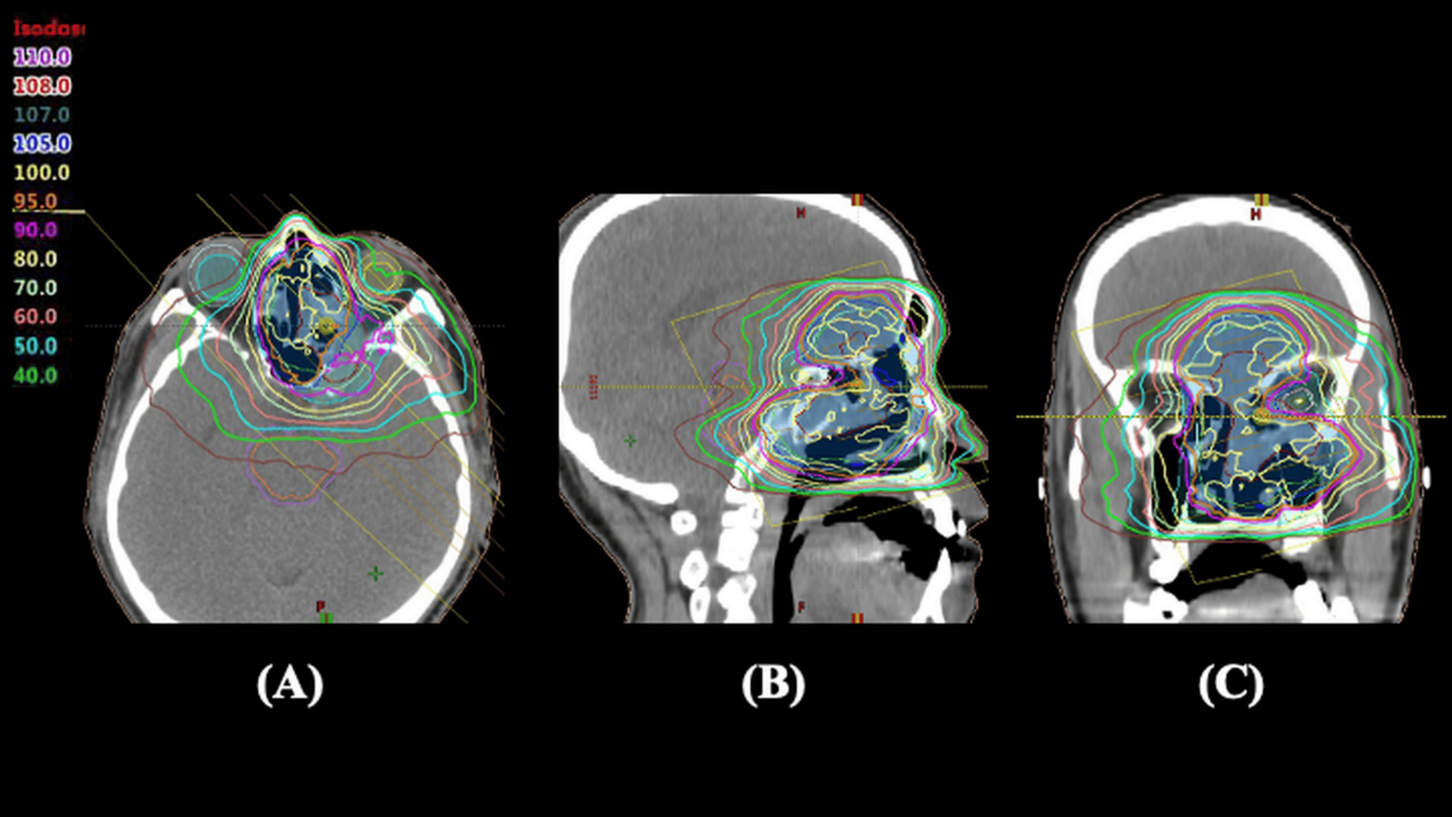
Dose distribution of intensity-modulated radiation therapy (IMRT) using a simultaneous integrated boost (SIB) technique Axial (A), sagittal (B), and coronal (C) CT images show the dose distribution. The gross tumor volume (GTV) is contoured in dark red, the clinical target volume (CTV) in lime green, and the planning target volume (PTV) in cyan. The left eyeball is delineated in yellow. Colored isodose lines represent percentages of the prescribed dose.
